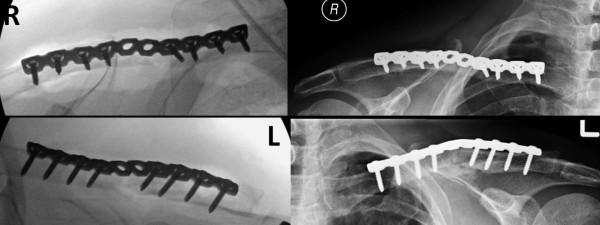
Intraoperative fluoroscopy films of bilateral clavicle fracture fixation in bridging technique (left panels), and follow-up radiographs at 6 months, demonstrating the bilateral healed fractures (right panels).
